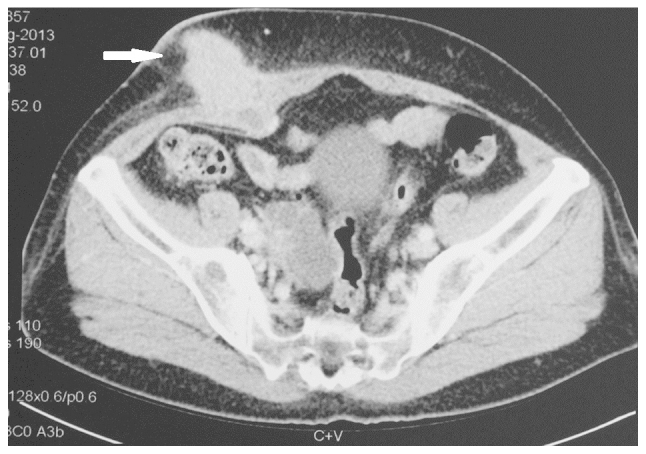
Computed tomography scan of a subcutaneous nodule (6.2×3.3 cm).
